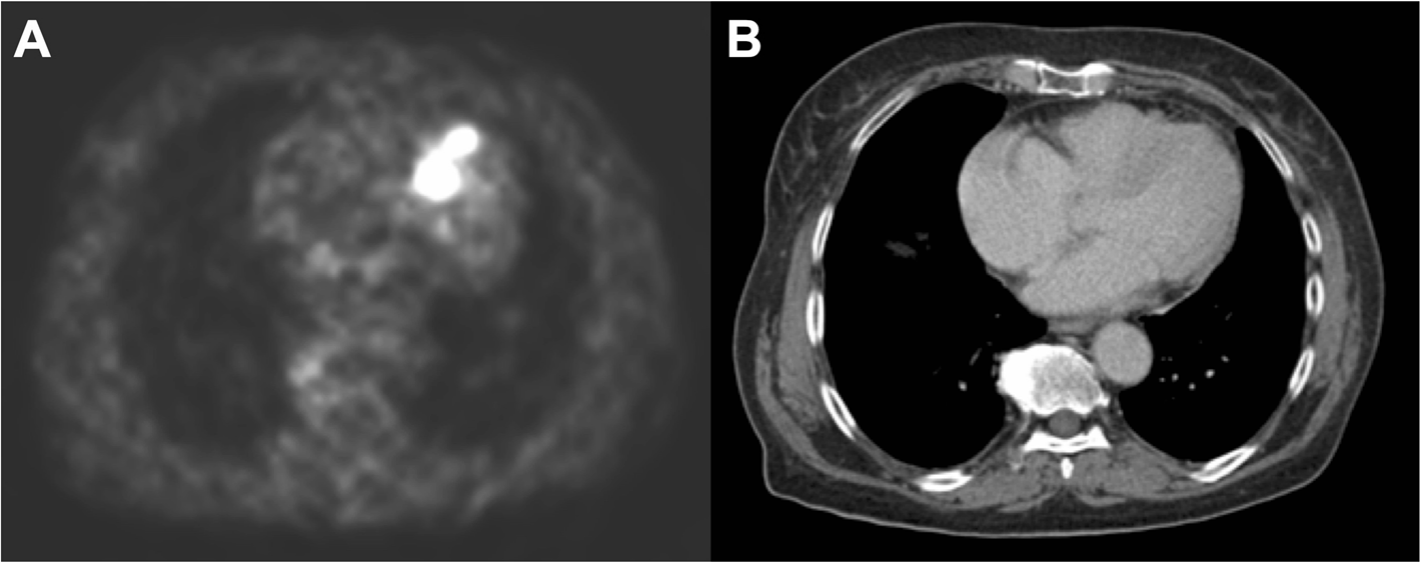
Patient example with a myocardial metastasis detected using 68Ga-DOTATATE PET (a) without evidence on CT (b). In a 74-year-old female patient with a G2 neuroendocrine tumor of the small intestine, 68Ga-DOTATATE PET (a) demonstrates strong focal uptake in the interventricular septum without a morphologic correlate on CT imaging (b). This uptake was observed throughout all follow-up examinations consistent with a myocardial metastasis
